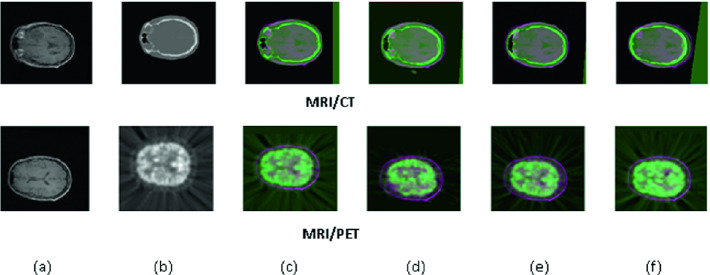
Examples of MRI/X multimodal registration: (a) MRI image, (b) X image, superposed images using (c) HM, (d) SPM (e) ITK-Snap, and (f) 3D Slicer.
